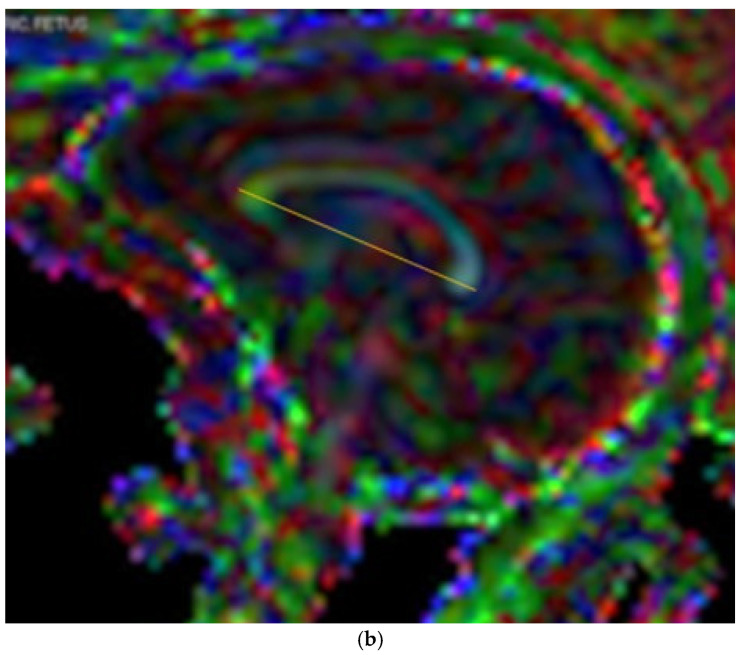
Mid-sagittal slice of the fetal brain in (a) T2-weighted image and (b) DTI with an example of fetal CC biometry measurement demonstrated by the yellow line. The images were taken from two different fetuses.
